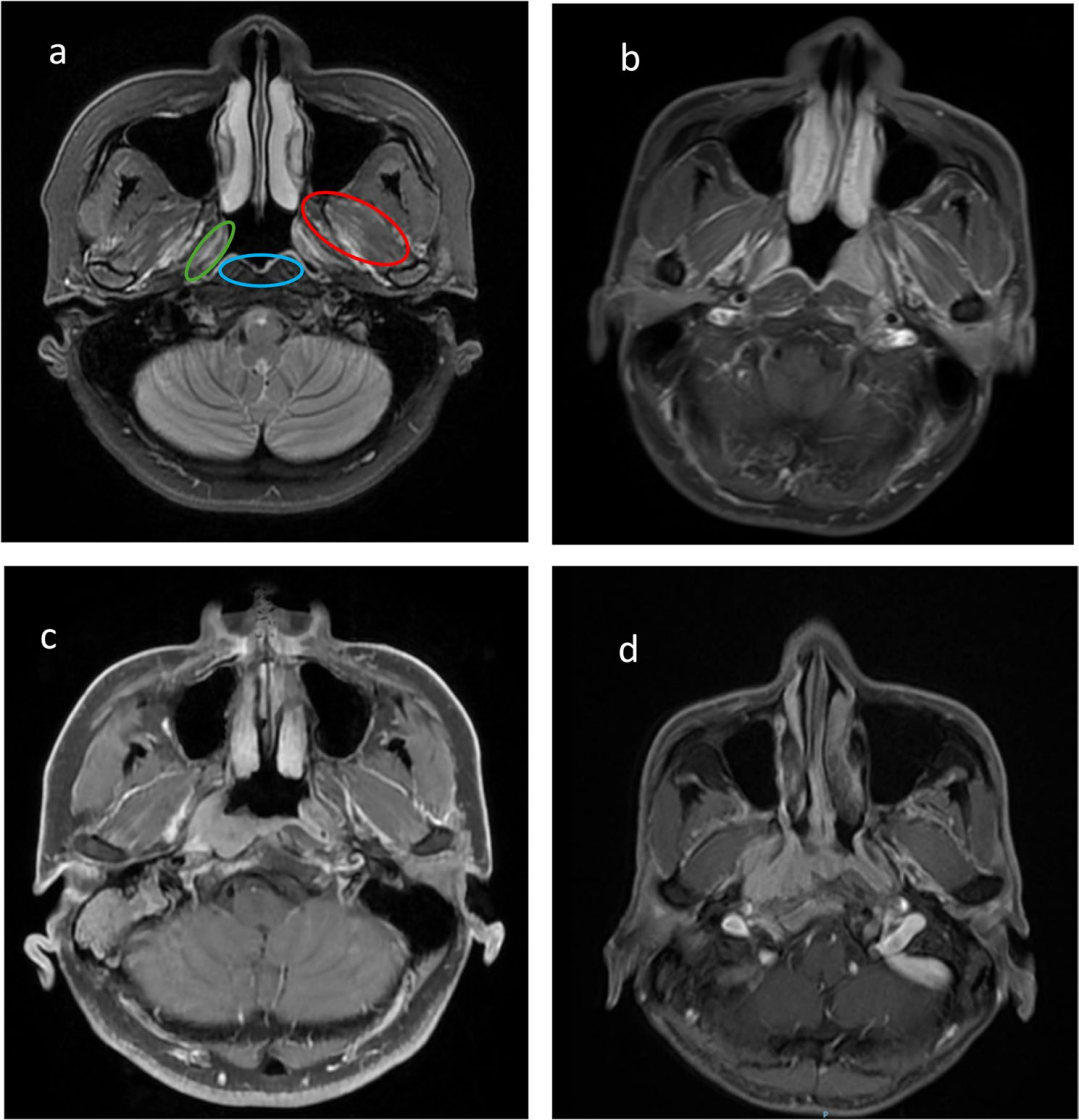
Representative magnetic resonance images (MRI) for different STI grades. a Axial T2-weighted MR image of normal nasopharynx displays the distribution area of STI. If the tumor invades the green, blue and red areas, they represent mild, moderate, and severe soft tissue invasion, respectively. b Contrast-enhanced T1-weighted images in a 42-year-old man. The nasopharynx lesions are accompanied only by LVP invasion (arrow), which is defined as mild STI. c Contrast-enhanced T1-weighted mages in a 48-year-old man with nasopharynx lesions extending to the prevertebral muscle (arrow), which is defined as moderate STI. d Contrast-enhanced T1-weighted images in a 56-year-old woman show the extensive invasion of LP (arrow), which is defined as severe STI. STI, soft tissue involvement; LVP, levator veli palatini; LP, lateral pterygoid
